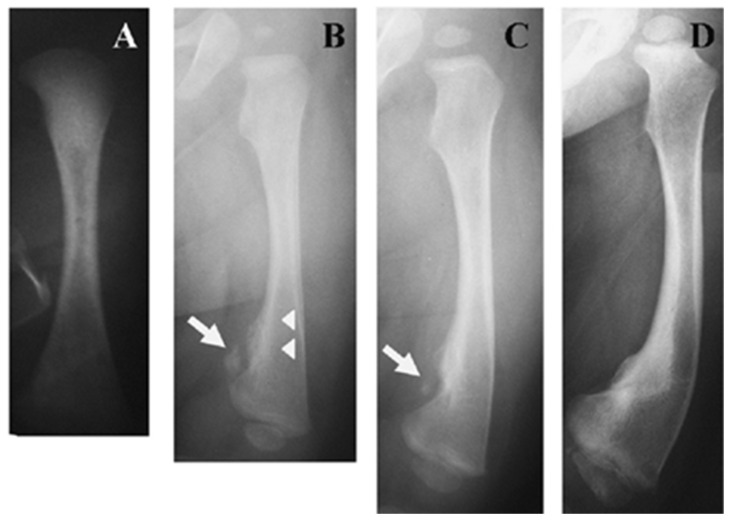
Radiographic progression of angular deformity in FFCD of the distal left femur. Reproduced from Tohoku J. Exp. Med. (Ando et al., 2008) [60] with permission from Tohoku University Medical Press. Anteroposterior radiograph of the left femur. (A) Two weeks after birth. Abnormal change was not apparent. (B) Ten weeks. A sclerotic change (arrowheads) with bony island (arrow) became apparent. (C) One year. The bony island became smaller (arrow) and varus deformity was apparent. (D) Two years. Varus deformity progressed up to 40 degrees.
